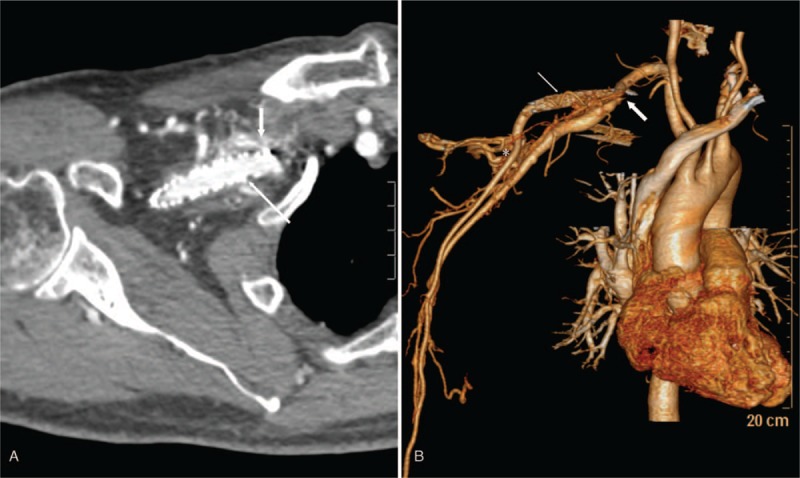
Computed tomographic angiography showed the stent in the subclavian artery (thin arrows in A and B), the occluded subclavian vein compressed by the stent (thick arrows in A and B), and the multiple arteriovenous malformations between the right subclavian artery and vein (asterisk).
